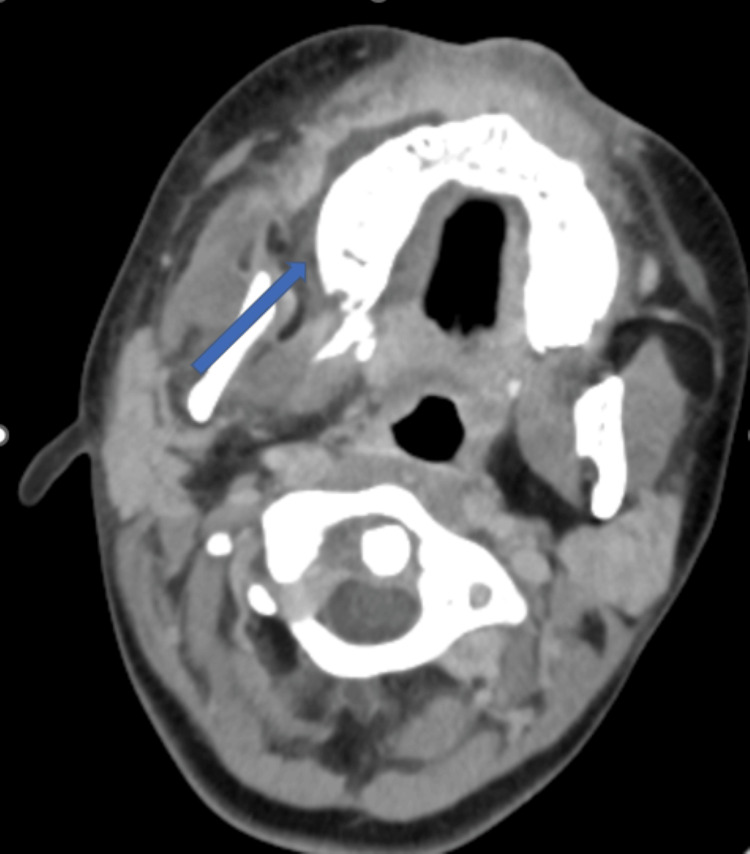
Axial CT image showing infiltration of the right jugal subcutaneous soft tissues (arrow) CT: computed tomography
